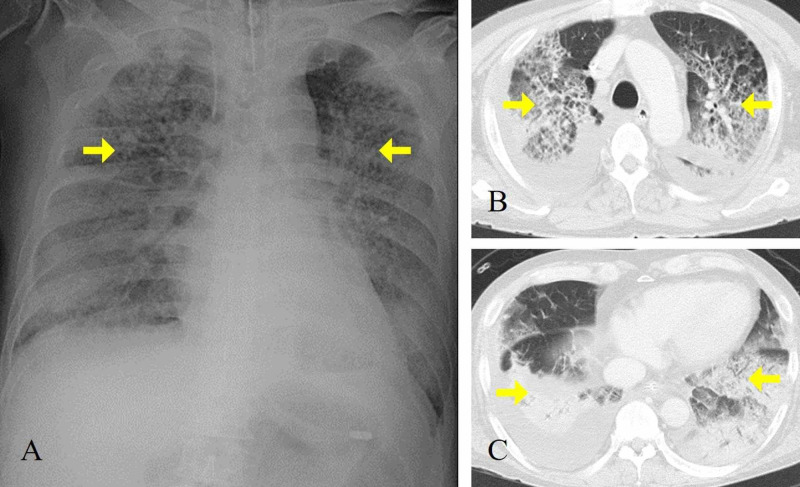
Chest X-ray and CT at ICU admission Chest X-ray (A) and CT (B, C) showing bilateral alveolar infiltrates with normal cardiac size consistent with acute respiratory distress syndrome.
